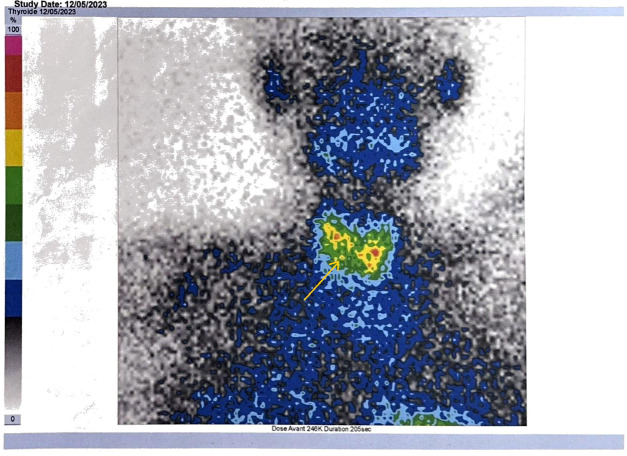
Parathyroid scintigraphy demonstrating a hyperfunctioning parathyroid gland.
